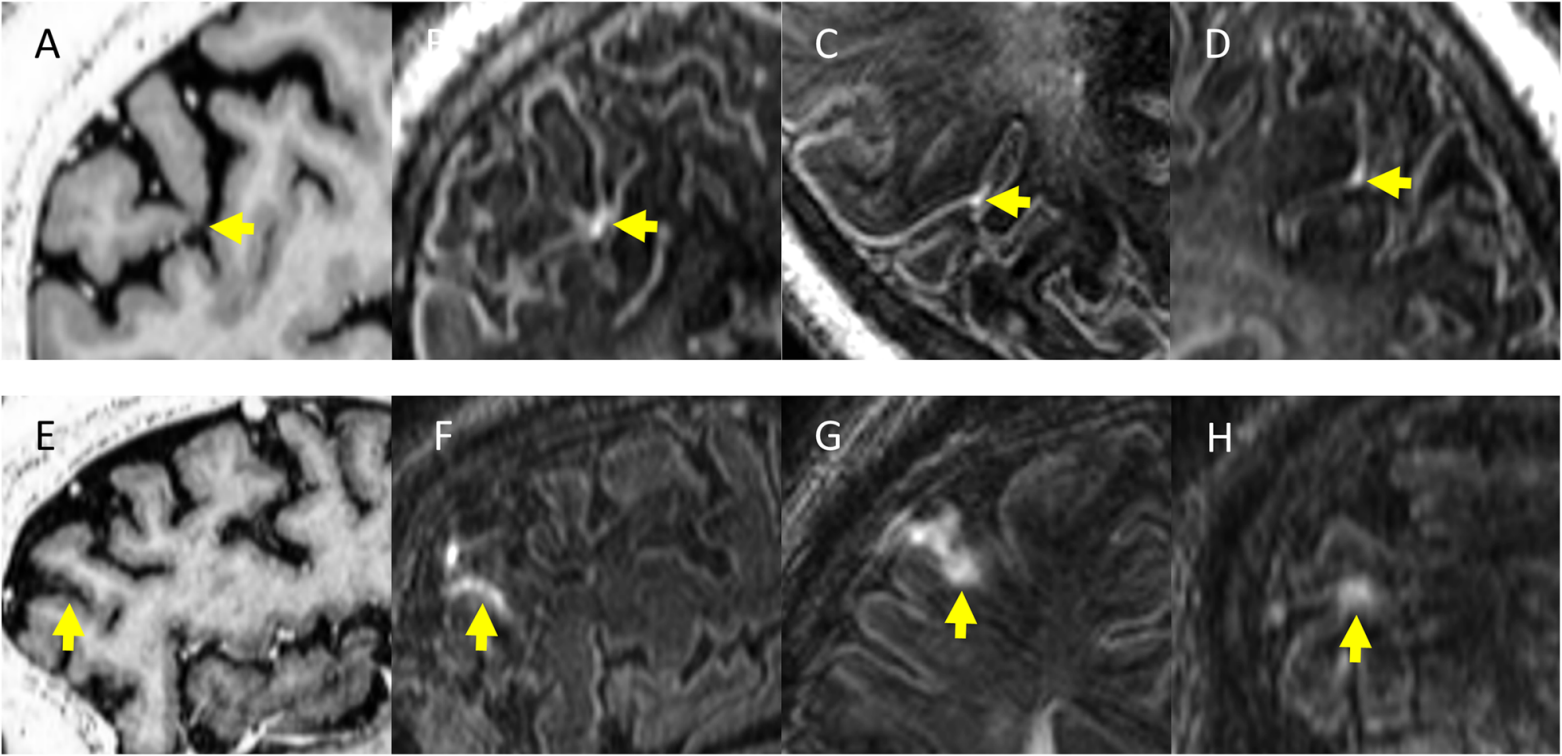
Examples of “spread/fill” type enhancing foci in multiple sclerosis. In the horizontal rows are displayed two different patients. Contrast-enhanced T1-weighted magnetisation-prepared two rapid gradient echo images (A, E), along with axial (C, G), sagittal (D, F), and coronal (B, H) slices from contrast-enhanced magnetisation-prepared fluid-attenuated inversion recovery images. The yellow arrows indicate the location of a spread/fill focus of leptomeningeal contrast enhancement in all the three planes and its expected location on T1-weighted magnetisation-prepared two rapid gradient echo images. Published with permission from *J Neurol* [53]
